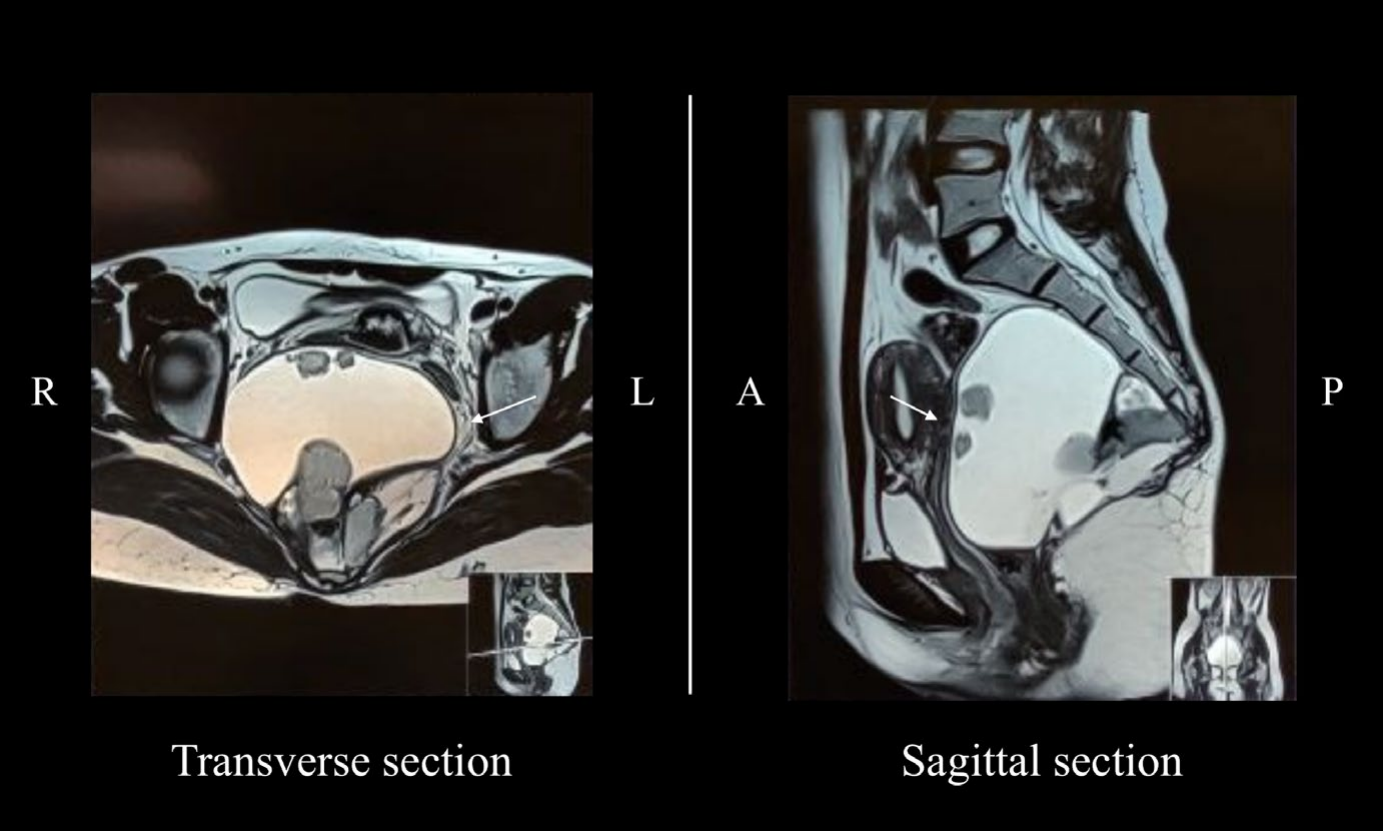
Magnetic resonance imaging of the pelvis showing a presacral lesion
